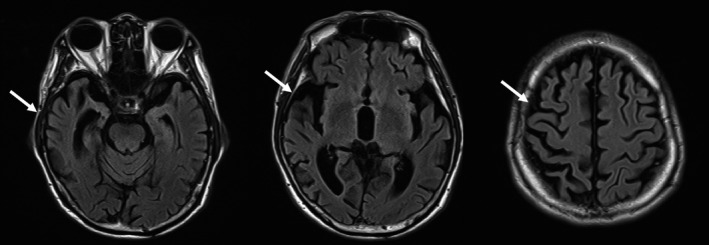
Brain MRI of the index patient. Brain MRI image, T2 FLAIR, showing atrophy in bilateral temporal, parietal, and frontal lobes, featuring asymmetric atrophy that is more severe in the right hemisphere (arrows). MRI, magnetic resonance imaging
